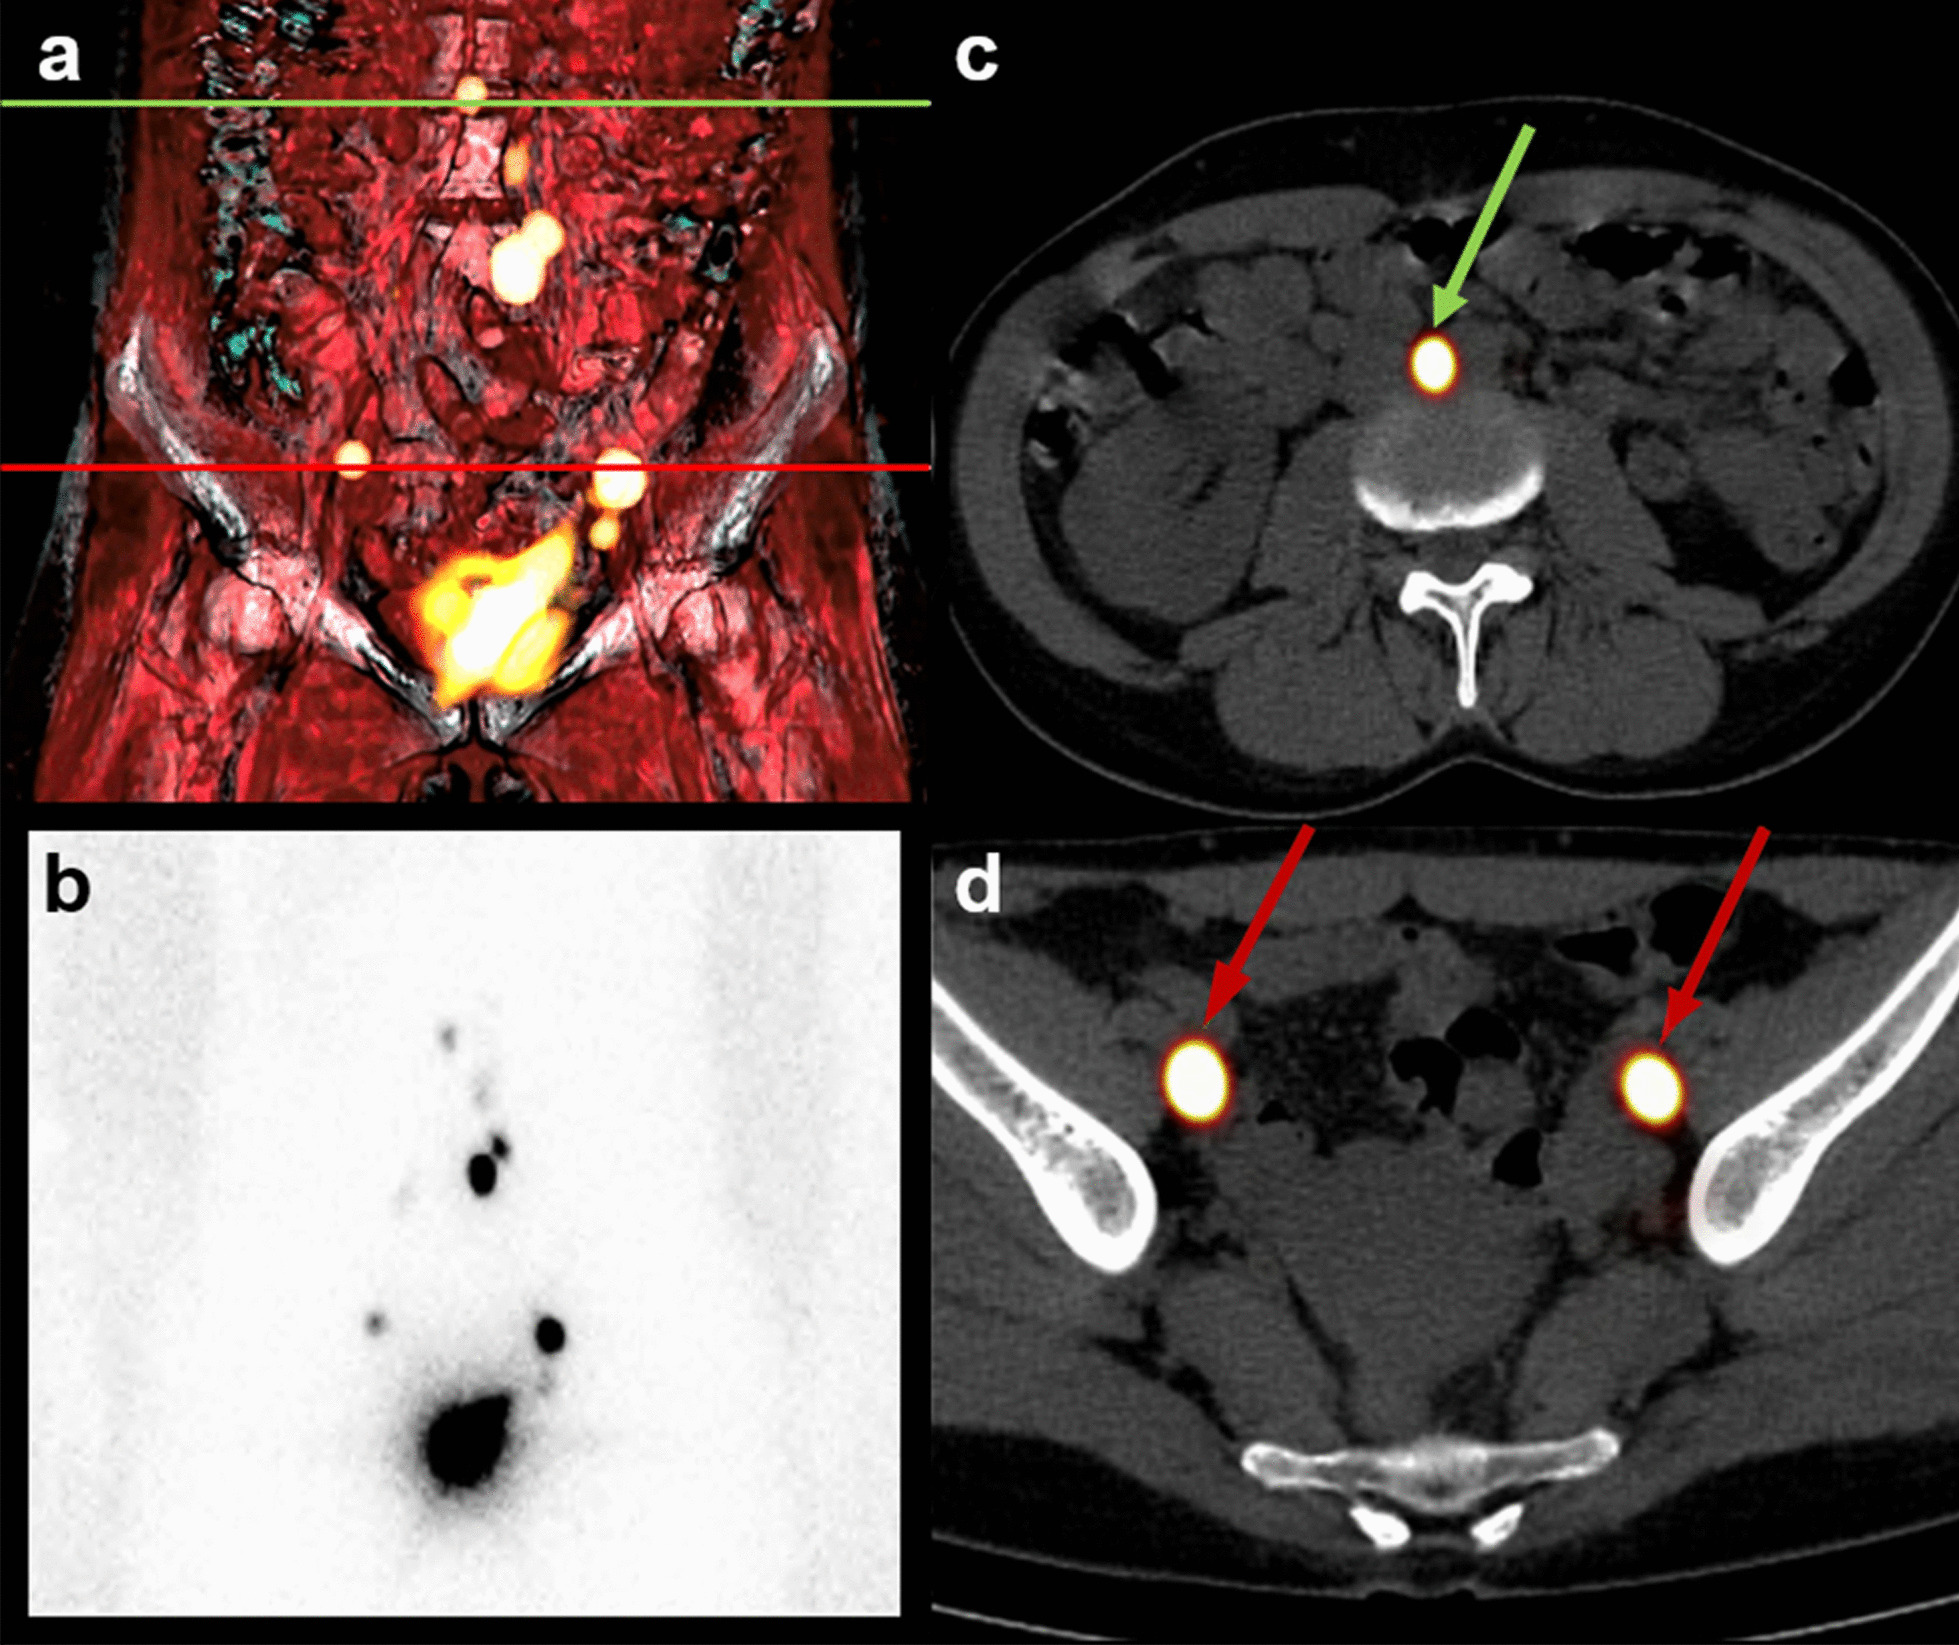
Planar and SPECT/CT lymphoscintigraphy performed 2 h after TUMIR injection of hybrid tracer (ICG-[^99m^Tc]Tc-nanocolloid). Volumetric reconstruction of SPECT/CT (a) and planar lymphoscintigraphy (b) reveal drainage to the bilateral pelvic and paraaortic regions. Axial fused SPECT/CT images showing radioactive SLNs corresponding to a paraaortic node (green arrow) (c) and bilateral external iliac SLNs (red arrows) (d). SPECT/CT: single photon emission computed tomography; TUMIR: transvaginal ultrasound-guided myometrial injection of radiotracer; SLNs: sentinel lymph node
